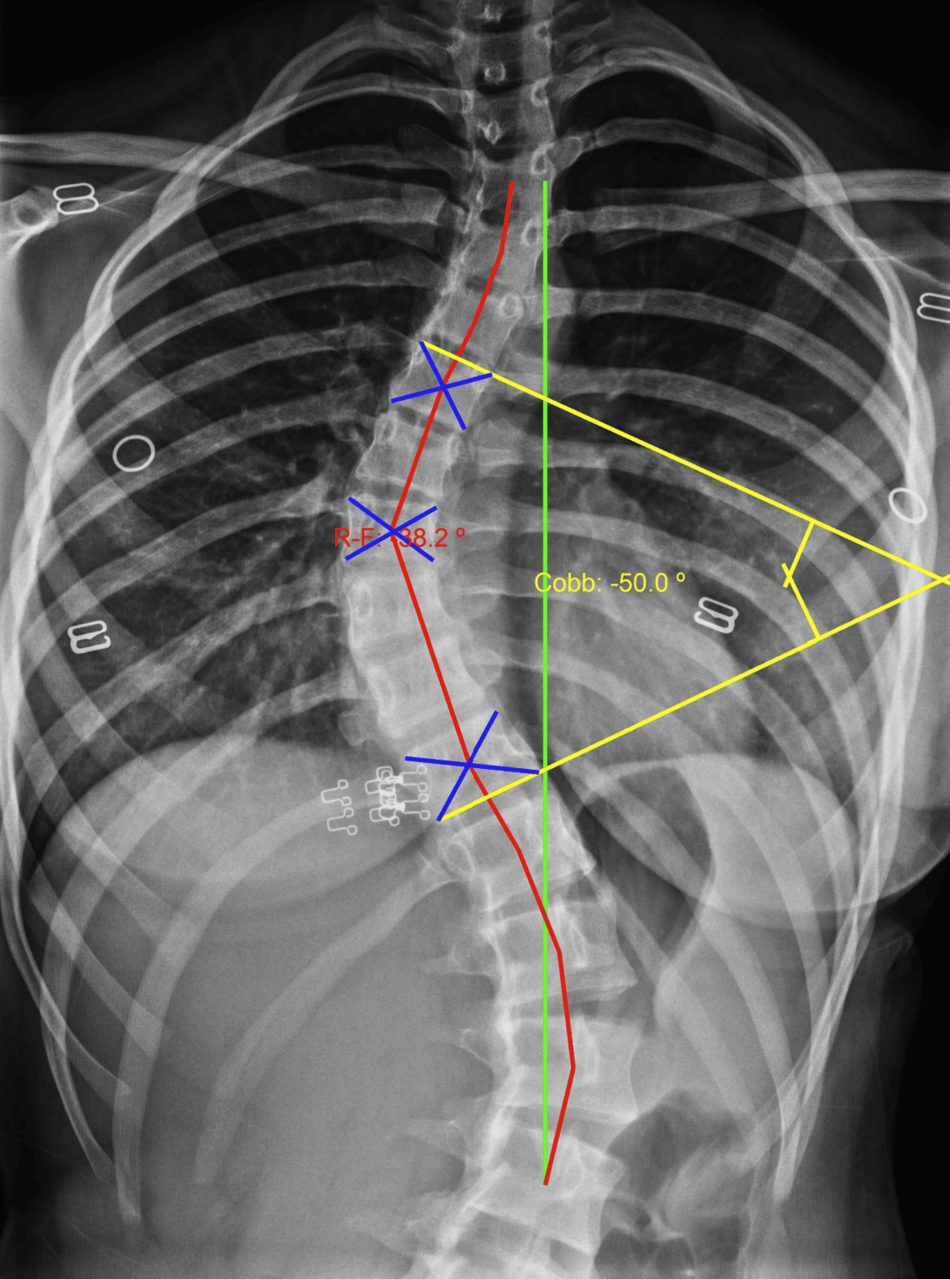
An AP thoracic/lumbar radiograph was taken at an FFD of 40 inches. The green line represents the normal spinal position, while the blue line indicates the center of the cross-section of the vertebrae. The red line represents the patient’s alignment, and the blue lines represent the projected centers of mass of the spine R-F: Risser-Ferguson method of analysis. Cobb: Cobb method of analysis with an apex at T8, a lower end vertebra at T11, and an upper measured vertebra at T6. FFD: focal film distance
